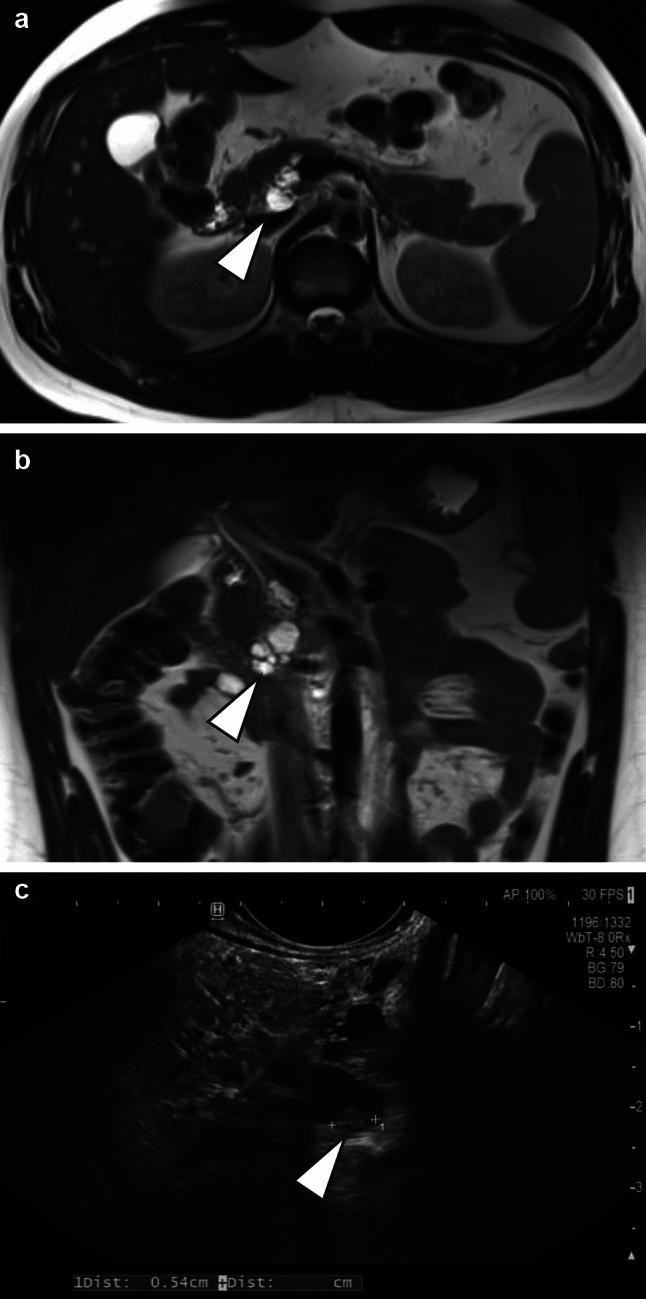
A Transverse T2-weighted MRI showing multiloculated cysts of the pancreatic head (arrowhead). B Coronal T2-weighted MRI showing multiloculated cysts of the pancreatic head (arrowhead). C Endosonography image with arrowhead indicating solid component
